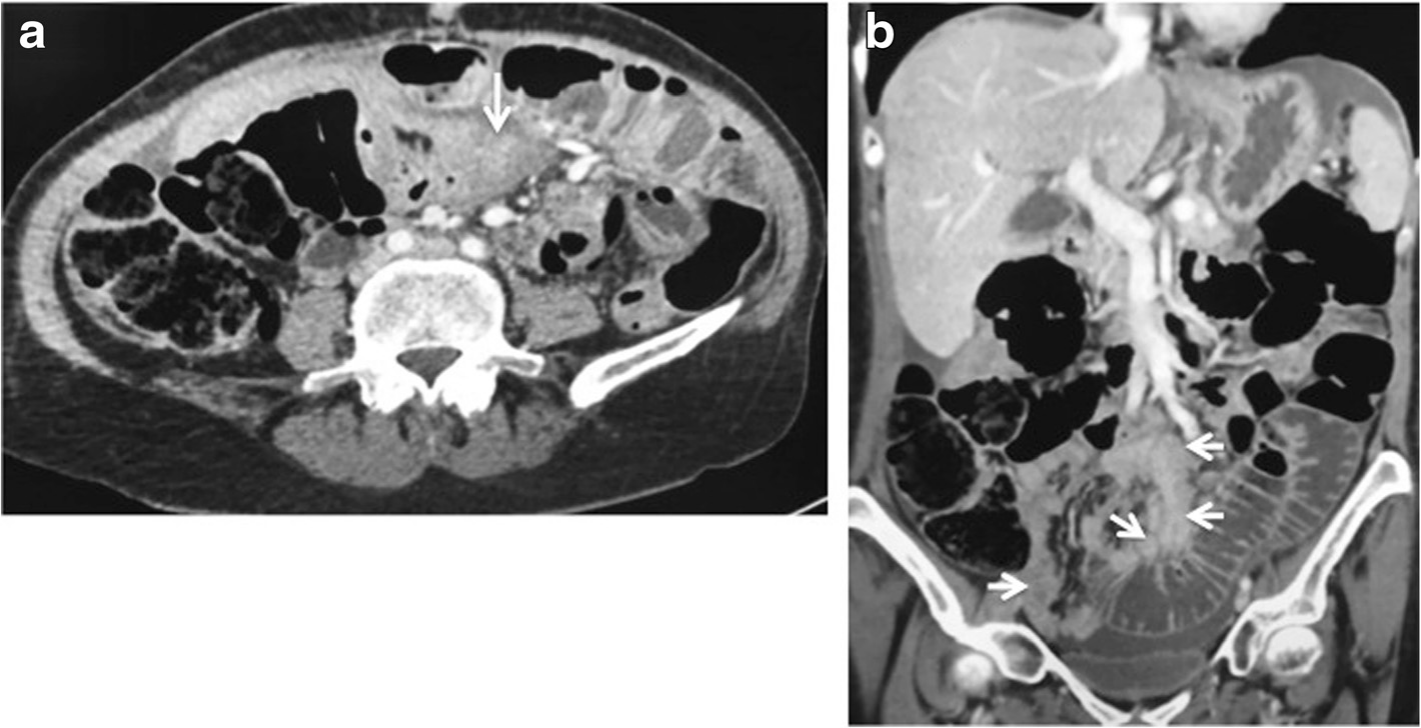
58 year old woman with stage IIIC undifferentiated carcinoma of the ovary. Axial (a) and coronal CT (b) shows extensive small bowel mesenteric disease (arrows) invading small bowel serosa and wall with resultant small bowel obstruction. Resection of this degree of mesenteric invasion would require sacrificing the entire small bowel
